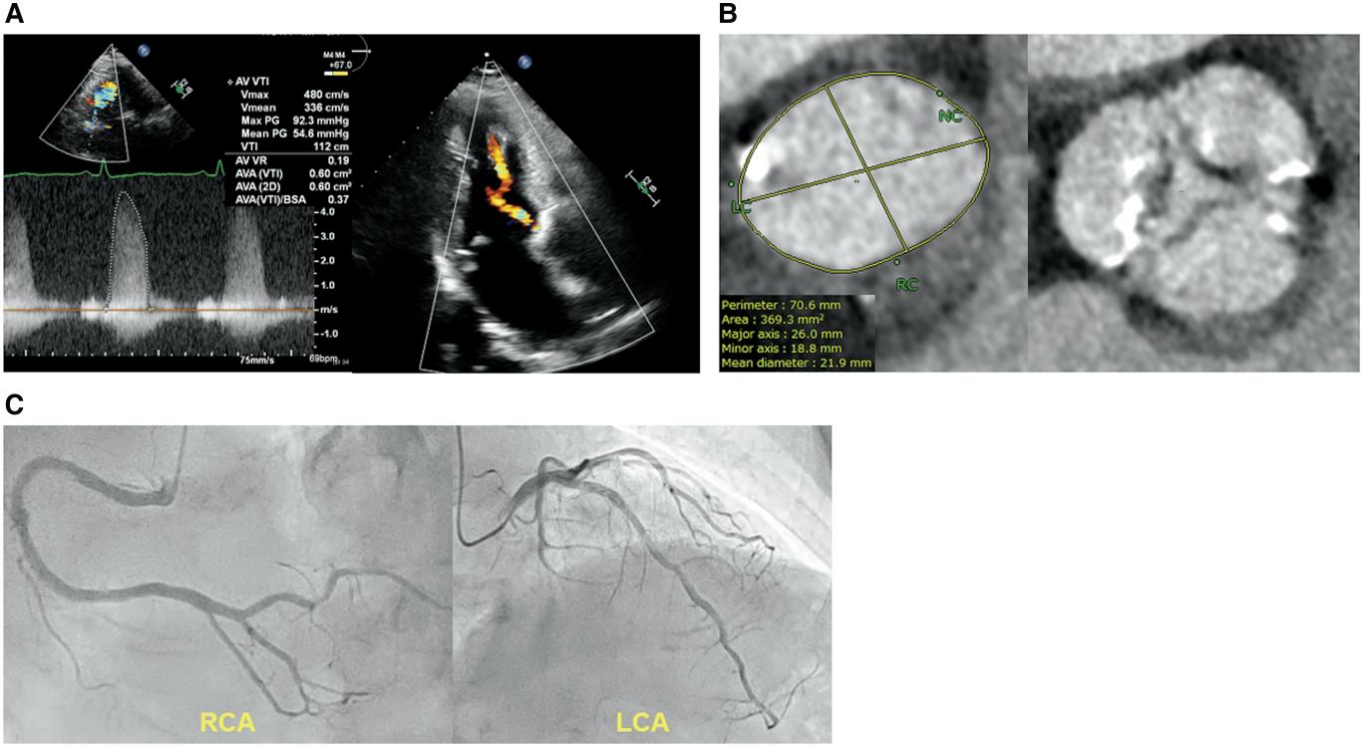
The preoperative imaging findings for the transcatheter aortic valve implantation procedure are presented. *(A)* Preoperative transthroracic echocardiography showed severe aortic stenosis and grade 1 aortic regurgitation. *(B)* Computed tomography revealed a tricuspid aortic valve with mild calcification. *(C)* Coronary angiography revealed no significant coronary artery stenosis.
